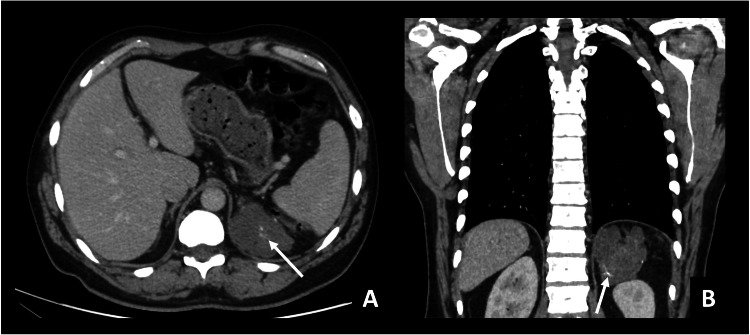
Chest CT with IV iodinated contrast (venous phase) Axial (A) and coronal (B) planes. Polylobulated mass in the left adrenal gland. It is homogeneous, with relatively low attenuation/mild enhancement (30 HU in portal venous phase). There are small calcification foci within the mass (arrows).
